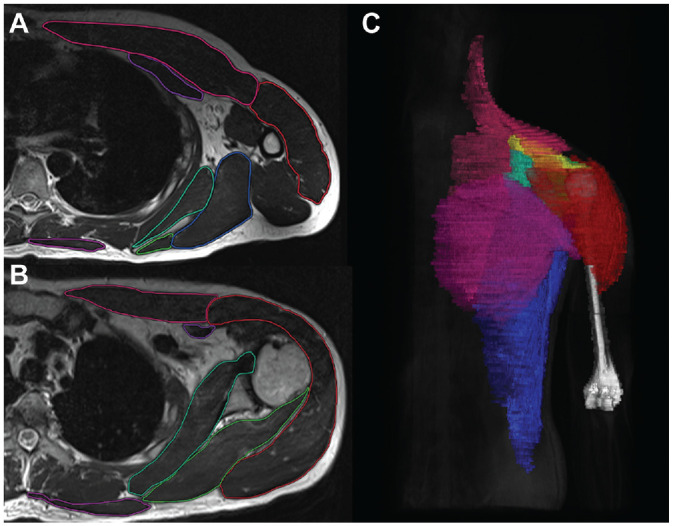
(A and B) Marking of shoulder girdle muscles on transverse slices. (C) Three-dimensional reconstructed model of measured muscles rendered from magnetic resonance imaging data. Deltoideus (red), pectoralis major (pink), subscapularis (turquoise), infraspinatus/teres minor (green), pectoralis minor (dark purple), latissimus/teres major (blue), trapezius (light purple), supraspinatus (yellow), and humerus (white).
